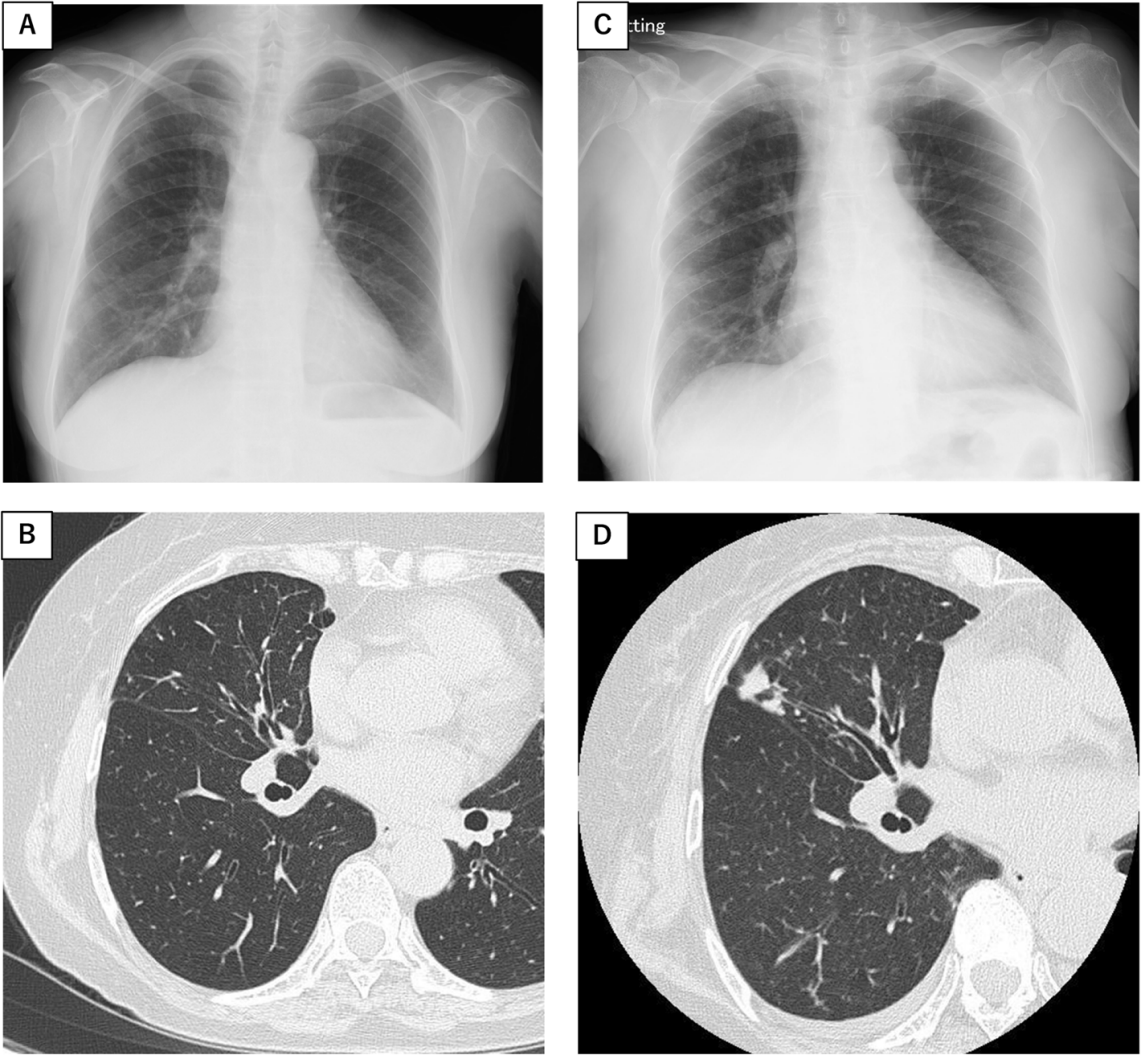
Partial progression of nodular shadow and bronchiectatic changes in right middle lung. The chest X-ray (a) and the chest computed tomography (b) performed when diagnosed with pulmonary MAC infection (2 years prior to current admission). The chest X-ray (c) and the chest computed tomography (d) performed at current admission
